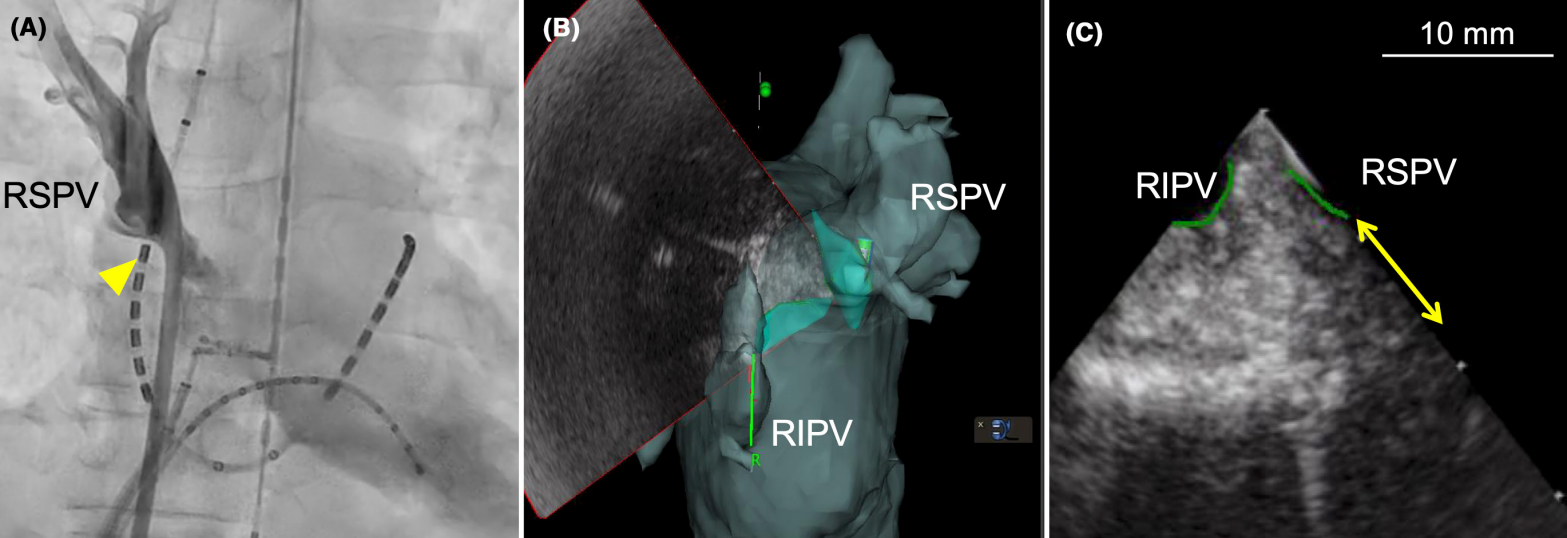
Acute PV stenosis after ablation. Selective PV angiography showed narrowing of RSPV. Intracardiac echocardiography demonstrated thickened and edematous wall of PVs.
